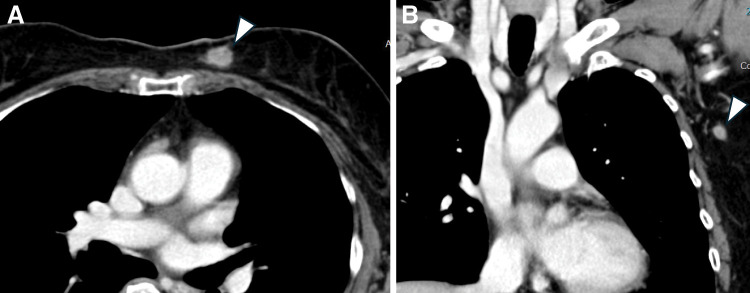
CT images. (A) Contrast-enhanced CT at approximately 28 months after treatment initiation showed an increase in breast mass size to 14 mm (white arrowhead). (B) An enlarged level I axillary lymph node was identified (white arrowhead), indicating progressive disease in the breast cancer.
